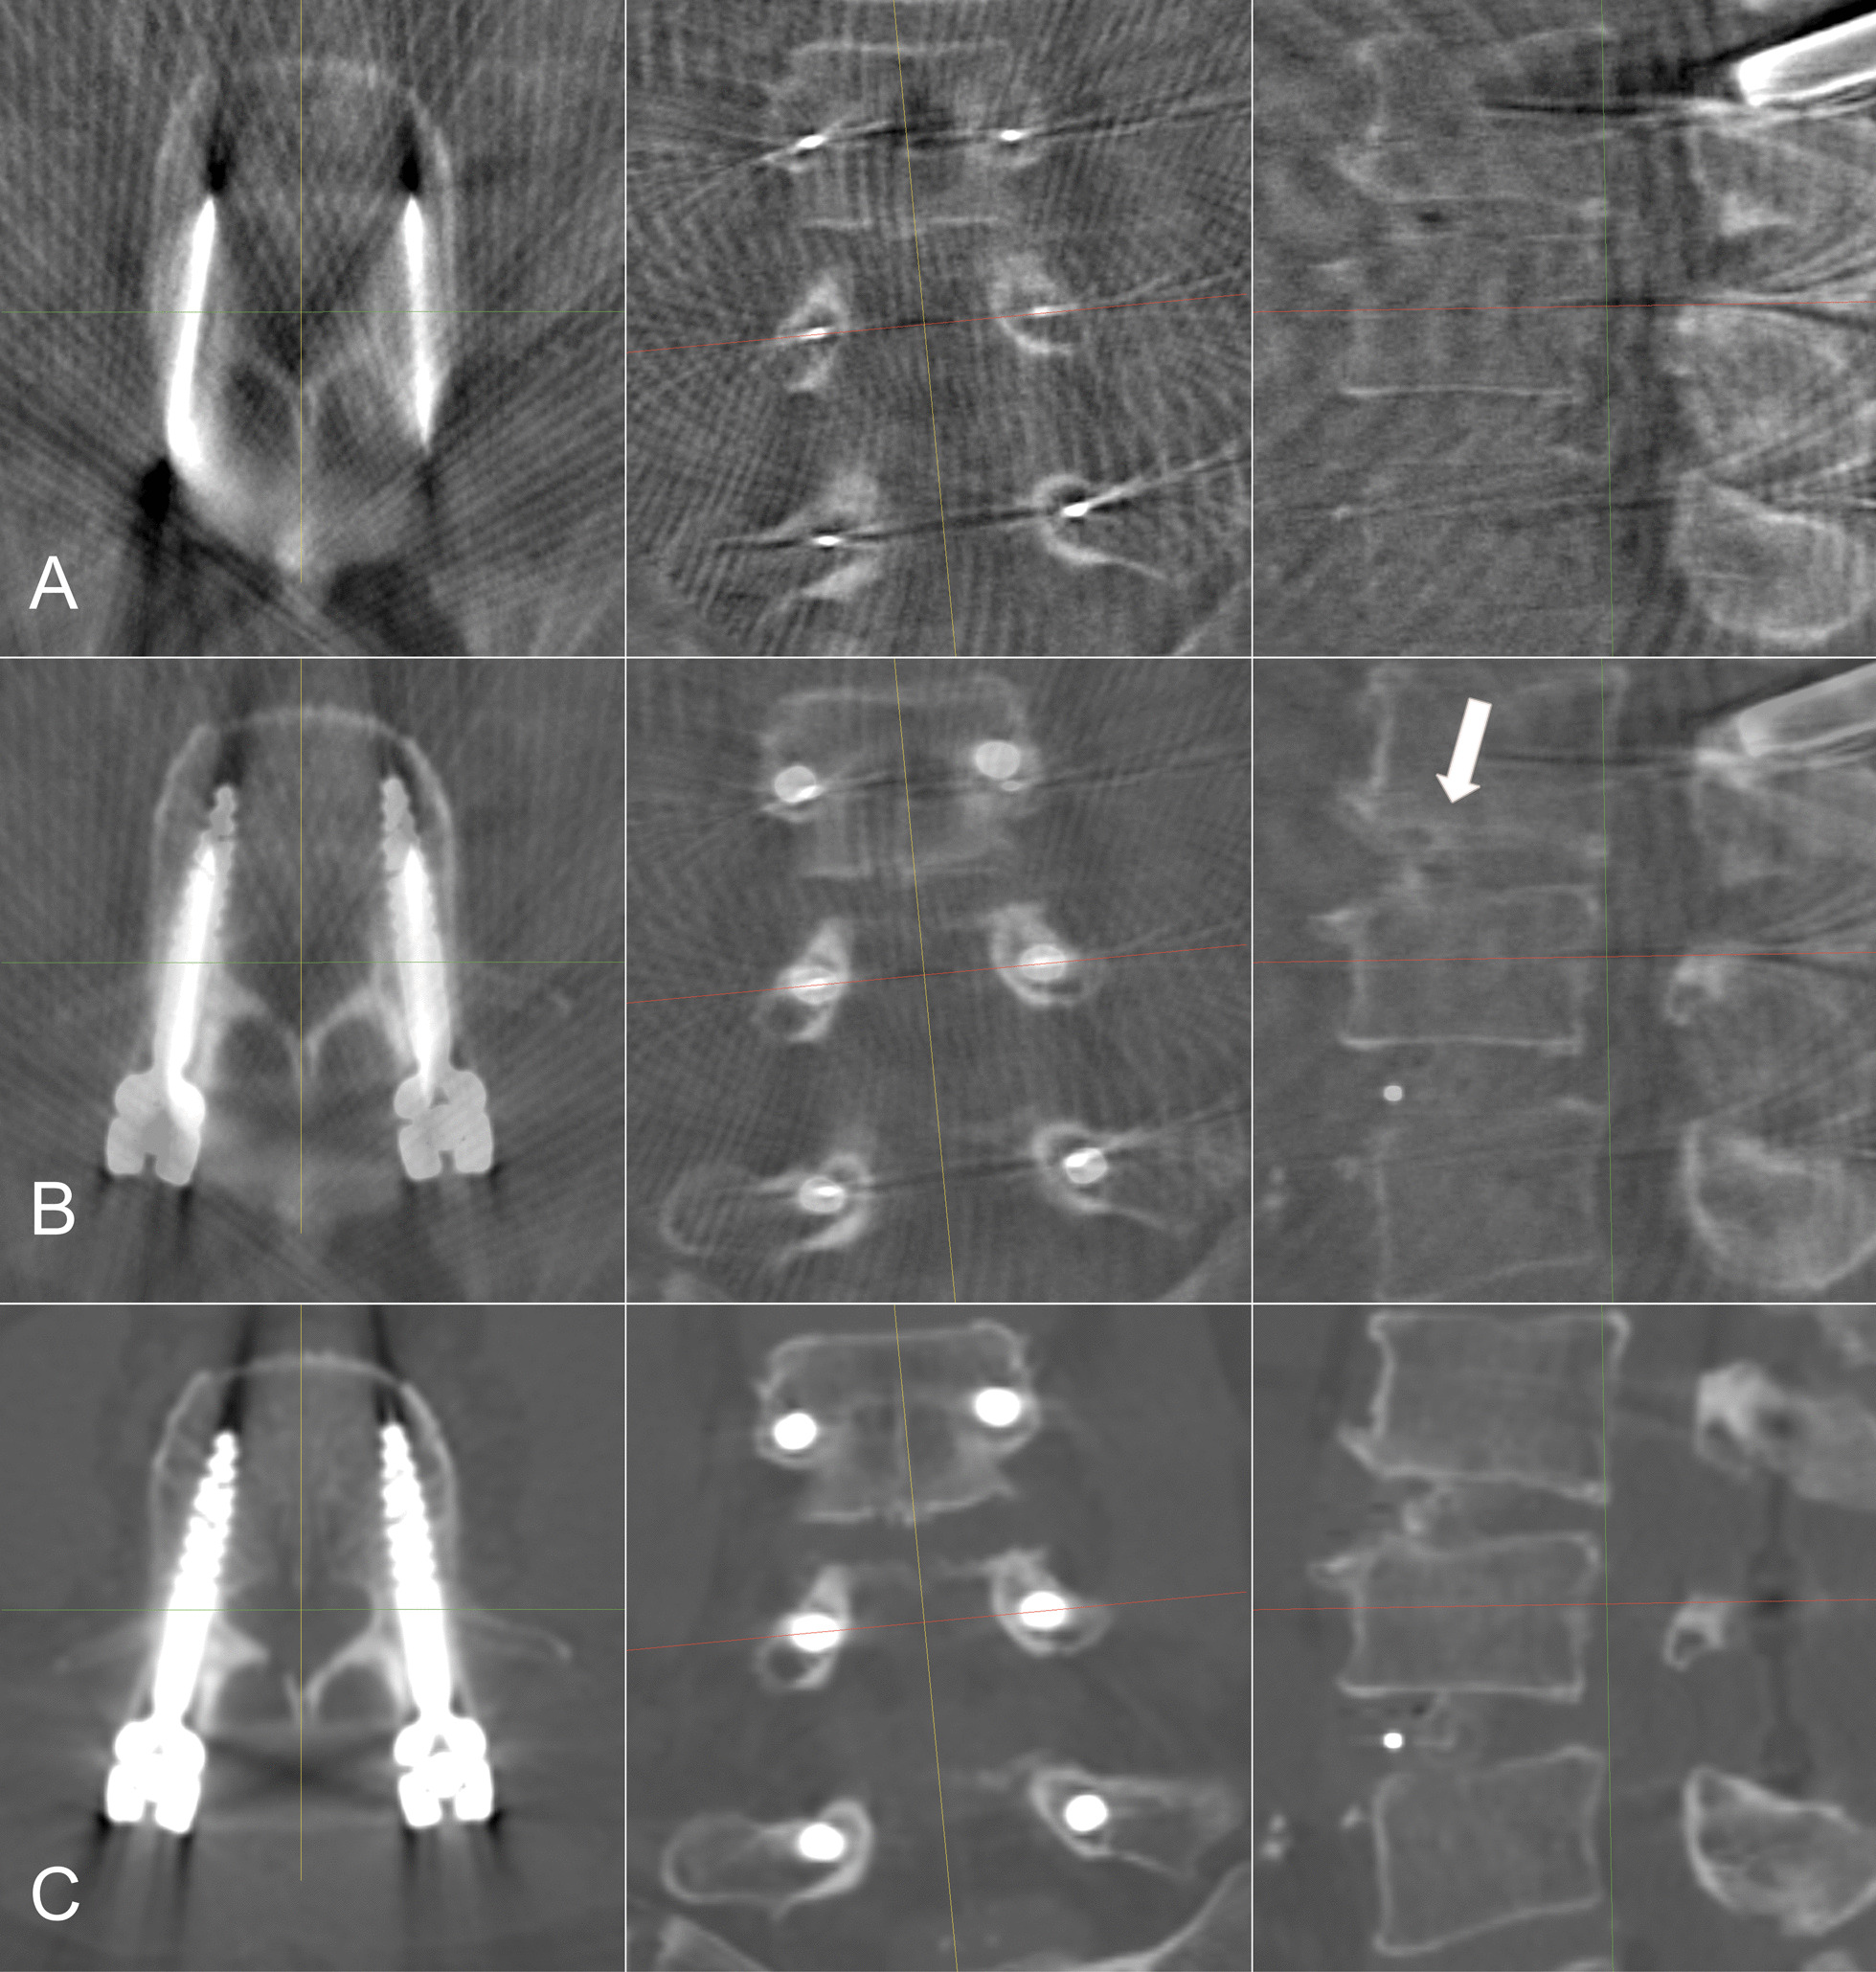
Image fusion result of IOCT and POCT, and assessment of the fusion. Each row shows three perpendicular reconstructed planes from a same CT. A: IOCT; B: Image overlay of IOCT and POCT. The target vertebra (centered) is fused. Note that the adjacent vertebra (white arrow) cannot be fused simultaneously because of the inter-segmental movement, thus it shows double edges of cortical bone; C: POCT
